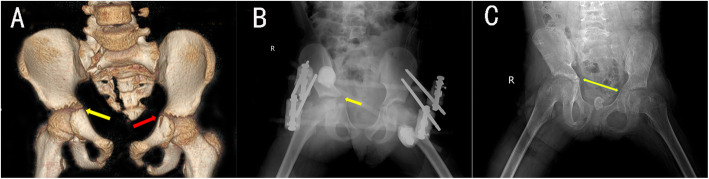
A 6-year-old girl (case A); Three-dimensional CT imaging of the pelvis revealed that the left suprapubic ramus fracture involved Y-shaped cartilage (red arrow), and the right Y-shaped cartilage gap widened (yellow arrow) (A); The postoperative X-ray showed that the pelvis was fixed with Orthofix external stent, and the right Y-shaped cartilage gap was still significantly widened (yellow arrow) (B). Seventeen months after the operation, the X-ray showed that the Y-shaped cartilage was partially closed (C)
